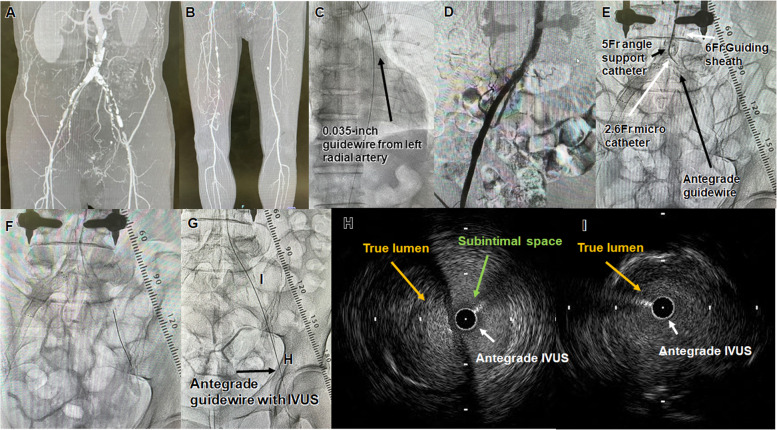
Case 1. A, B Preprocedural enhanced computed tomography images. C The transradial approach. The black arrow shows the 0.035-inch guidewire used to introduce the guiding sheath. D Control angiography showed a total occlusion of the left common iliac artery. E A 0.014-inch guidewire with a 5Fr angled support catheter and a 6Fr guiding sheath was advanced into the chronic total occlusion lesion. The white arrow on upper right shows the tip of the guiding sheath. The black arrow on upper left shows the 5Fr angled support catheter. The white arrow on lower left shows the 2.6Fr microcatheter. The black arrow on lower right shows the antegrade guidewire. F An antegrade guidewire was advanced to the distal external iliac artery (EIA). G The black arrow shows the antegrade guidewire with intravascular ultrasound (IVUS). H IVUS findings in the distal EIA. The white arrow shows the antegrade IVUS in the subintimal space. The yellow arrow shows the true lumen. The green arrow shows the subintimal space. I IVUS findings in the proximal EIA. The white arrow shows the antegrade IVUS. The yellow arrow shows the true lumen
